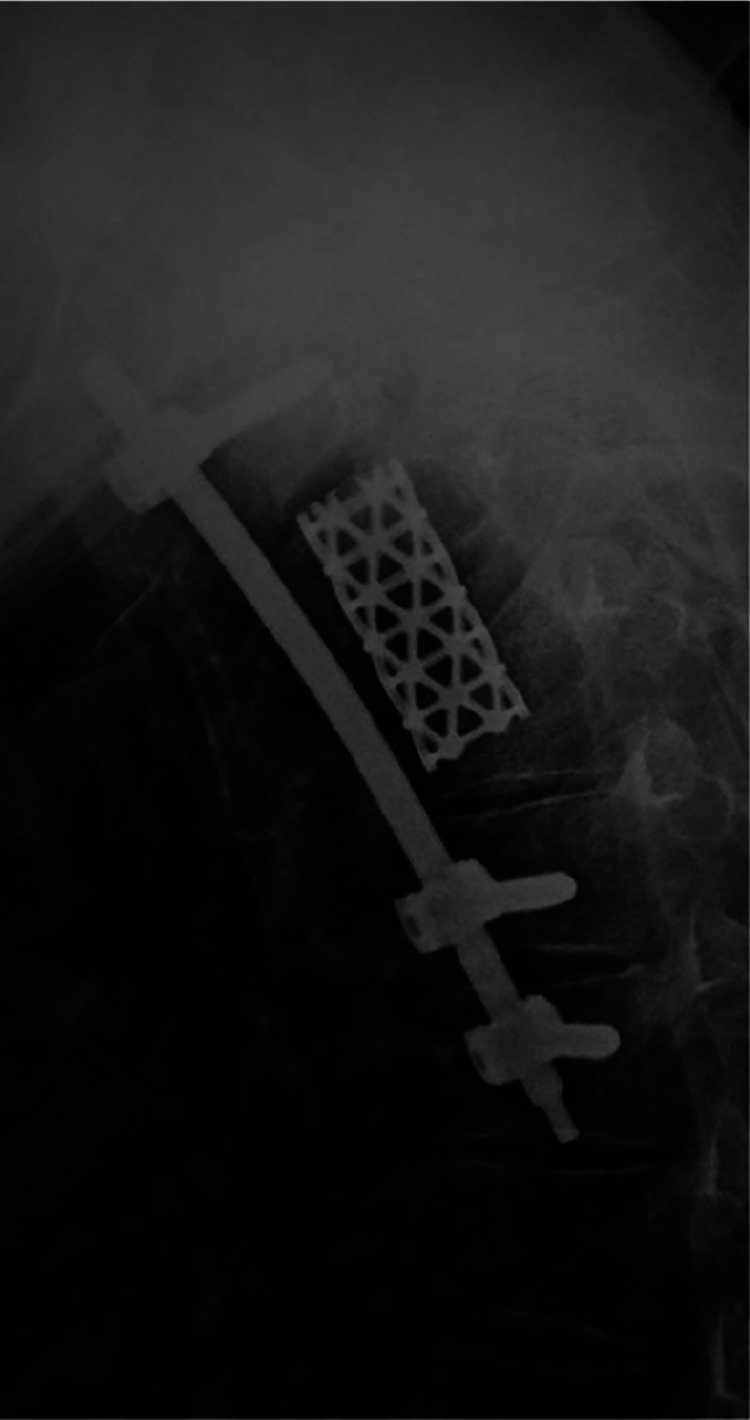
Postoperative lat x-ray.
